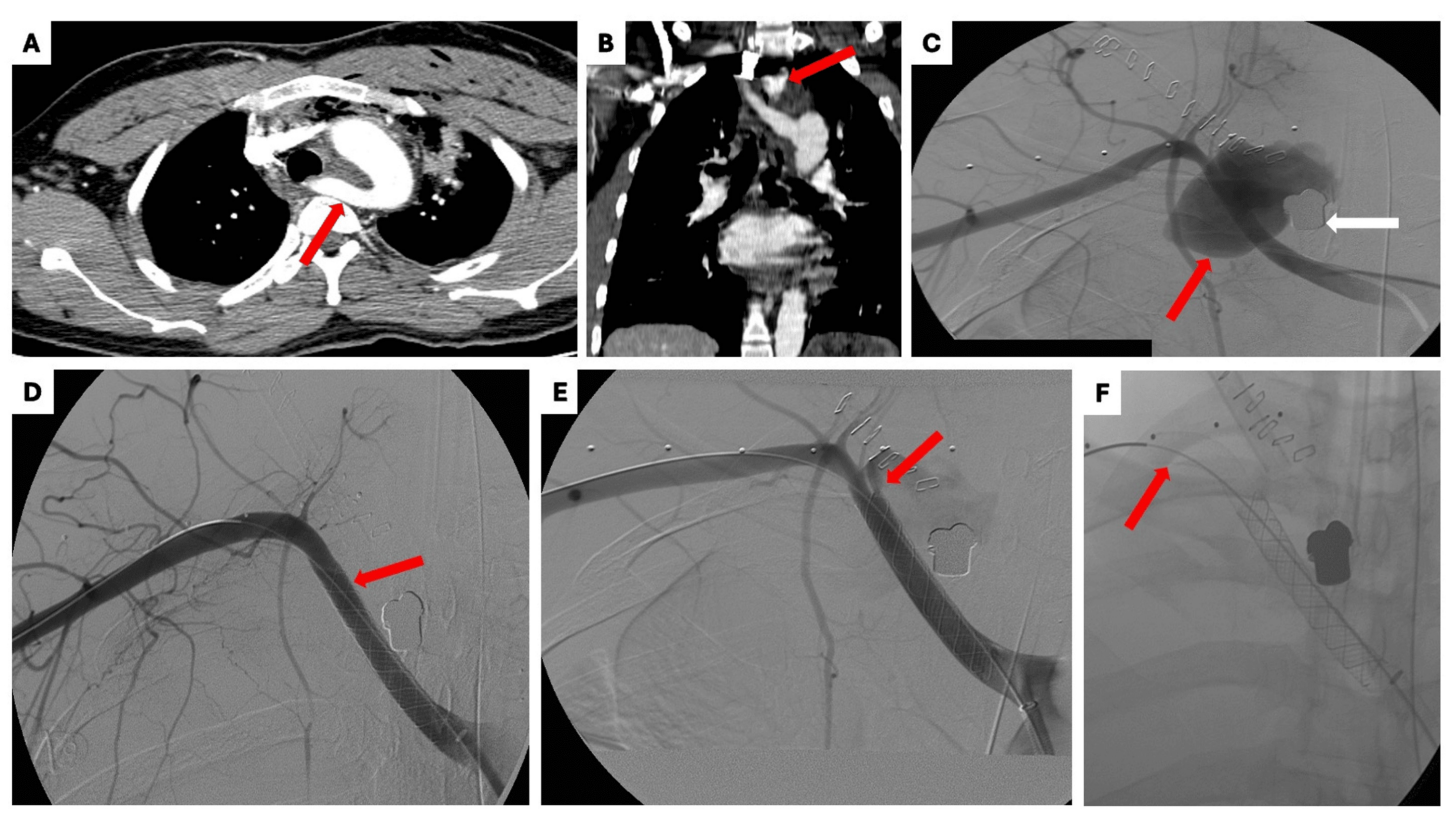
Emergent computed tomography angiogram (CTA) and postoperative imaging (A) Axial CTA with intravenous contrast at the level of great vessel origins demonstrating aberrant right subclavian artery (ARSA) (red arrow); (B) Coronal CTA of the chest demonstrating contrast-filled sac along the ARSA with irregular borders suggestive of pseudoaneurysm (red arrow); (C) Digital subtraction angiogram confirming the contrast-filled sac along the ARSA consistent with pseudoaneurysm (red arrow). Also, note the ballistic fragment adjacent to the sac (white arrow); (D) Covered stent was placed to exclude the pseudoaneurysmal sac (red arrow); (E) Immediate post-operative angiogram demonstrating type I endoleak along the distal aspect of the stent (red arrow); (F) Additional covered stent (red arrow) was placed to treat the endoleak while sacrificing the ostia for the right vertebral, thyrocervical trunk, and internal mammary arteries.
